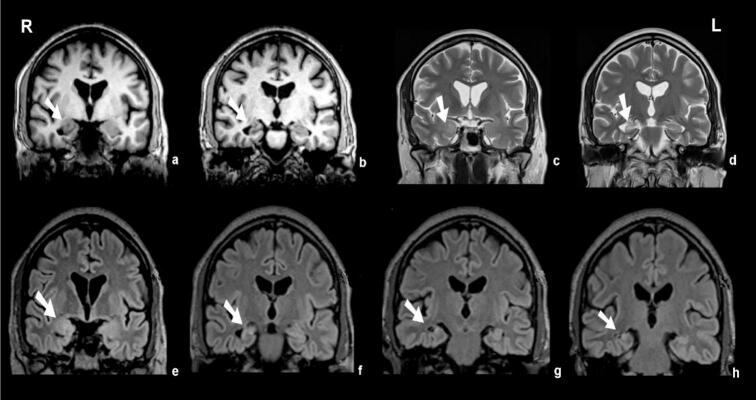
Coronal preoperative MR images demonstrating right mesial temporal abnormalities. T1-weighted images (a, b), and T2-weighted images (c, d), FLAIR (fluid-attenuated inversion recovery) images (e, f, g, h), obtained through the amygdala and the head of the hippocampus show right hippocampal atrophy with T2/FLAIR hyperintensity consistent with hippocampal sclerosis, accompanied by patchy signal changes in the right amygdala (arrows). R = Right L = Left.
